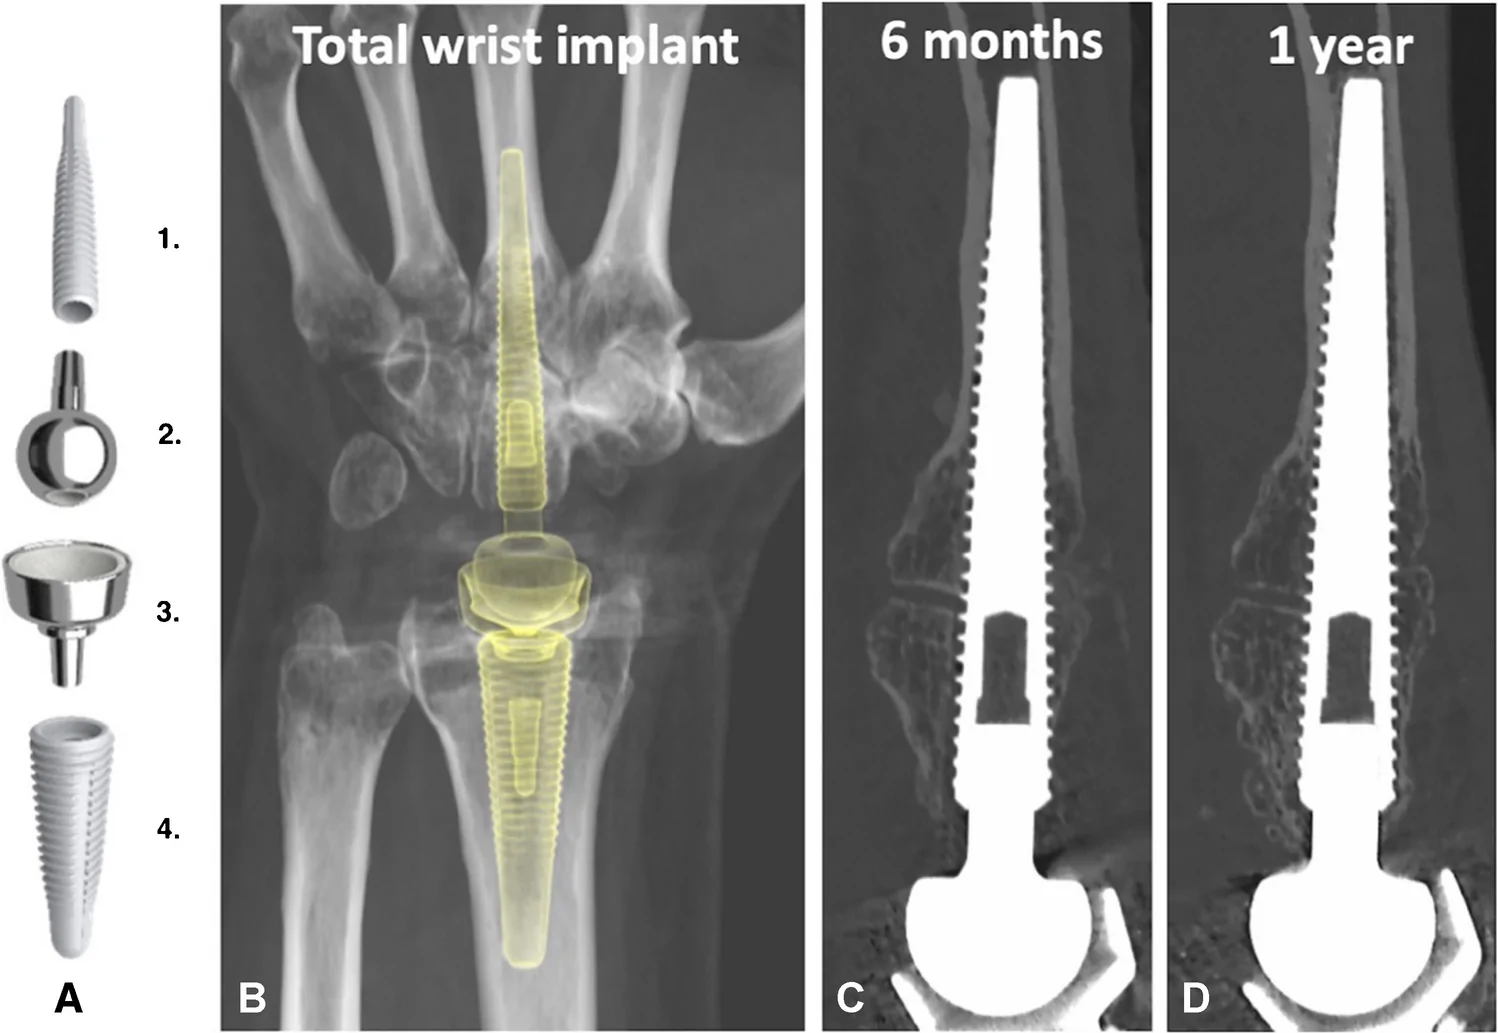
A The Motec Wrist arthroplasty with four separate modular parts: (1) distal screw, (2) ball, (3) polyethylene-coated socket, and (4) proximal screw, that can be changed to finesse the tension of the final implant position. B 3D reconstruction of a PCD-CT scan 1 year after implantation. Clinical examples of PCD-CT images of a fixed implant after 6 months (C) and 12 months (D), respectively
